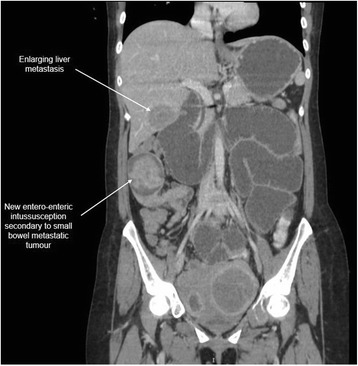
Subsequent coronal CT showing new entero-enteric intussusception and enlargement of liver metastasis
